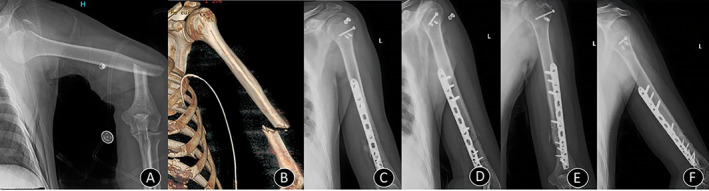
Case 3. (A) A plain radiograph showing dislocation of the shoulder with fractures of the greater tuberosity and humerus shaft. (B) A three‐dimensional reconstruction of a CT scan after reduction of dislocation. (C) and (D) Immediate postoperative X‐rays showing the humerus fracture fixed with locking compression plate and greater tuberosity fracture fixed with cannulated compression screws with rotator cuff tears repaired with suture anchors. (E) and (F) Four‐month follow‐up X‐ray showing union at fracture sites with reduced shoulder.
